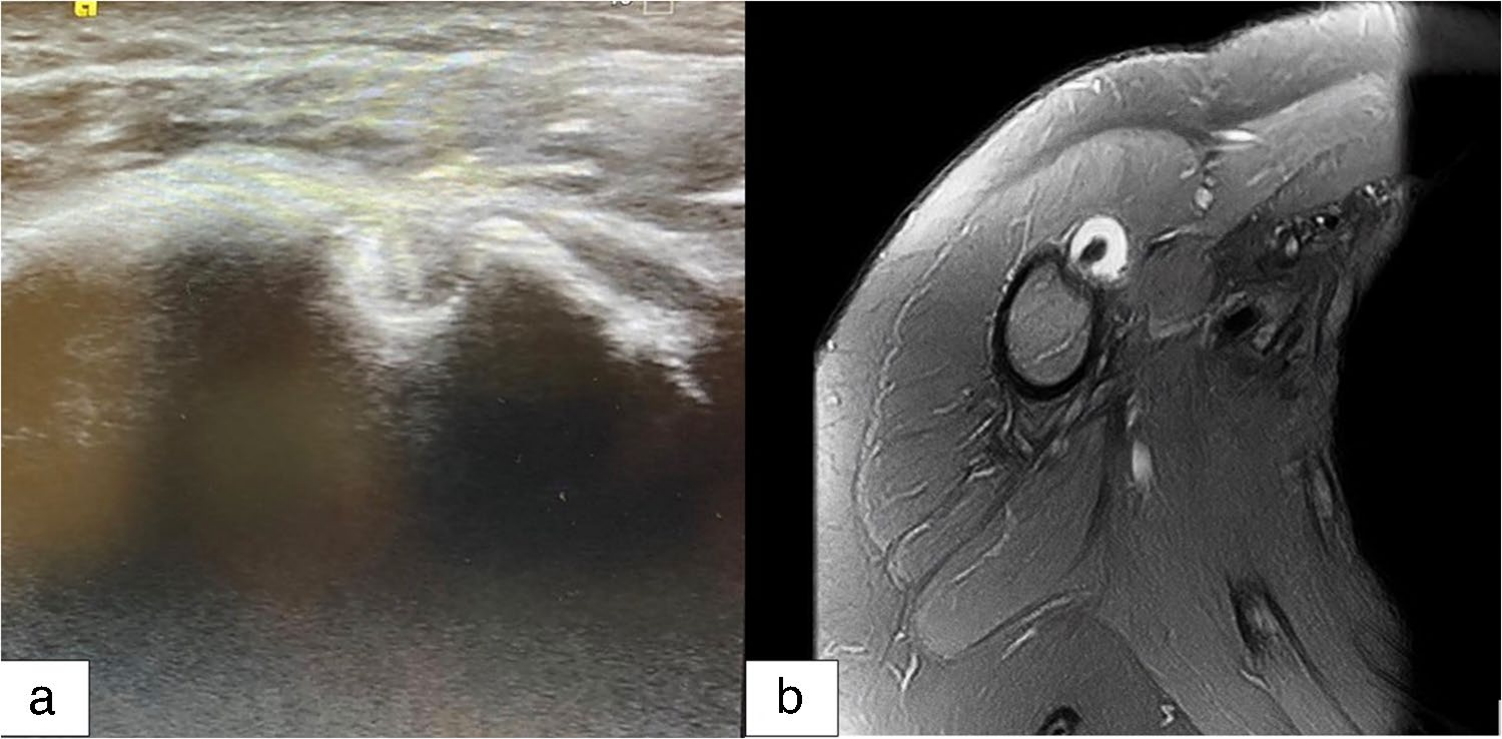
(a) Preoperative axial ultrasound image showing long-head biceps tendinopathy. (b) Preoperative axial T2-weighted MRI demonstrating increased signal within the LHBT consistent with tendinopathy
